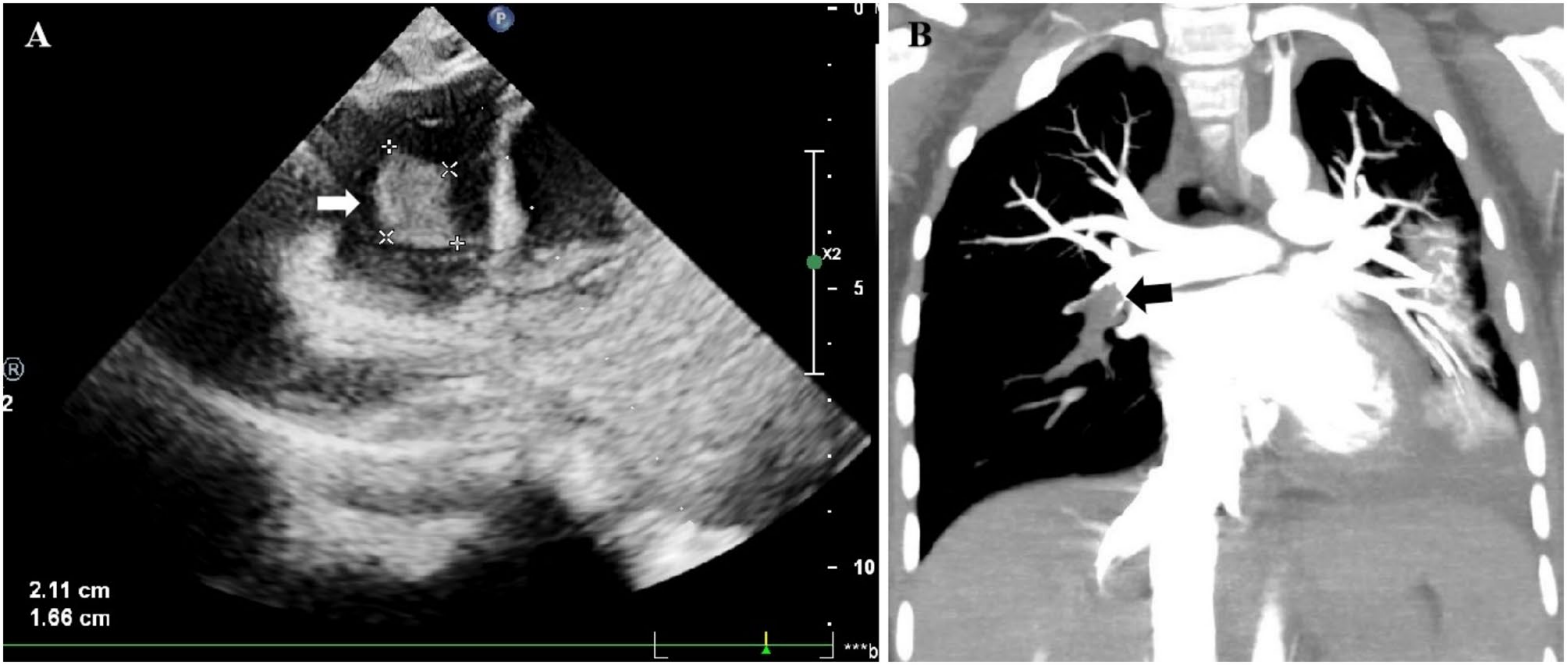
A, Echocardiography revealed a wall-attached mass (indicated by the white arrow) in the right ventricle. B, Enhanced Chest CT demonstrated a low-density filling defect in the lower right pulmonary artery (indicated by the black arrow), suggestive of pulmonary thrombosis
